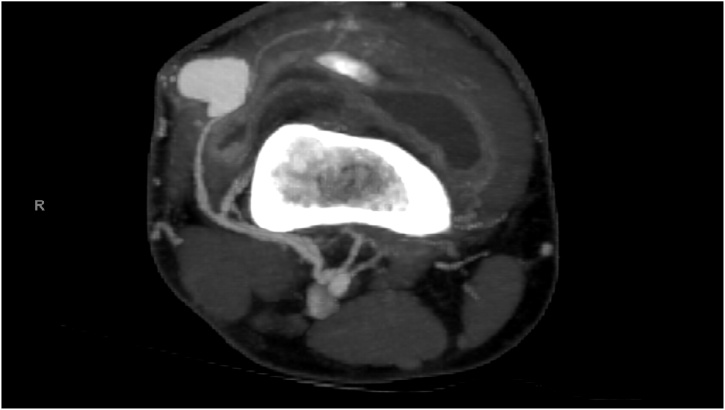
CTA showing the pseudoaneurysm. There is still a little edema due to the procedure. Axial plane of CTA showing the early opacification of pseudoaneurysm with contrast in arterial phase. Note the synovitis in the knee joint (post-operative aspect).
